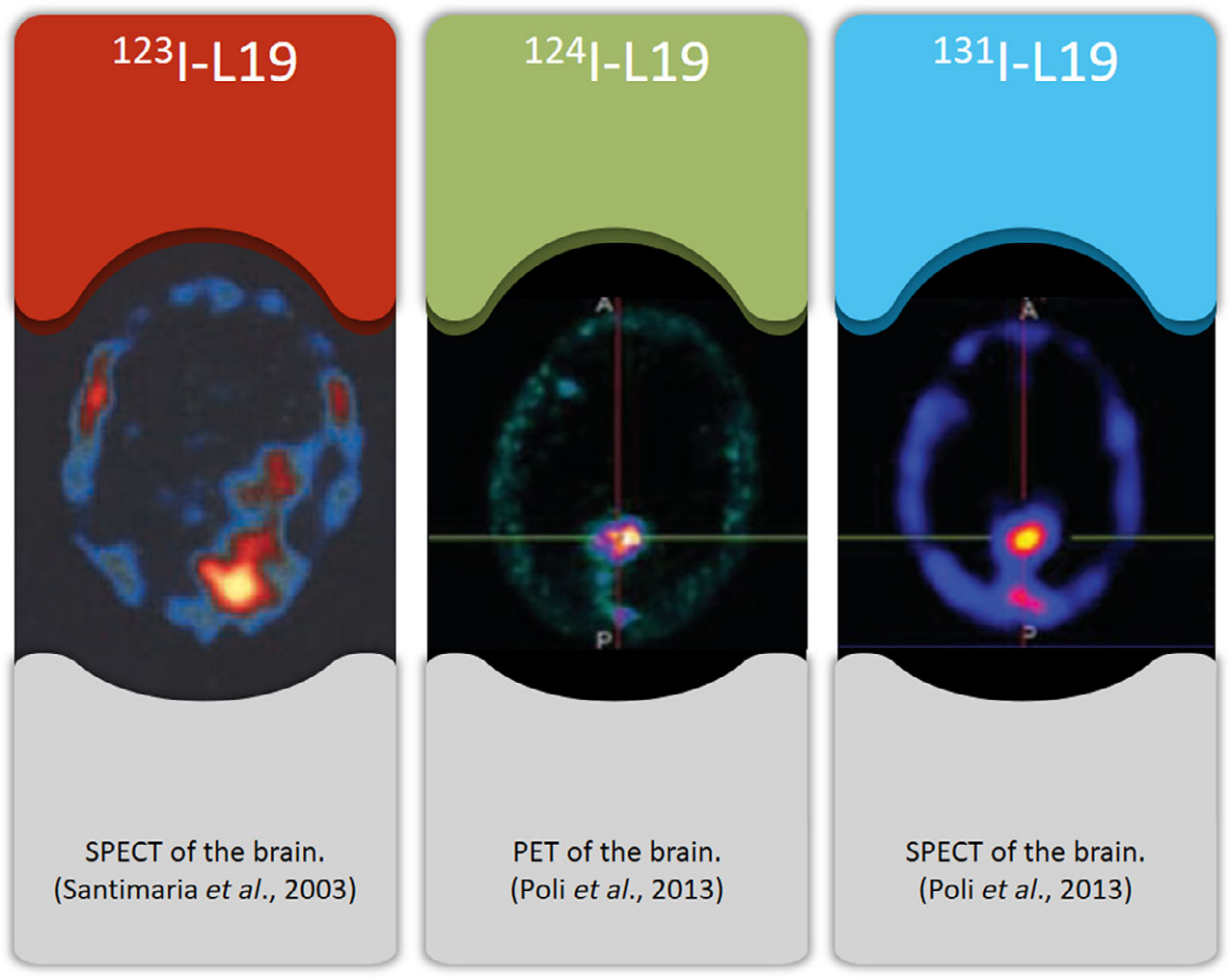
Methods to visualize ED‐B noninvasively in a patients' metastatic brain lesion. Left: SPECT scan where red/orange areas indicate uptake of ^123^I‐L19, middle: PET scan where purple/yellow area indicates uptake of ^124^I‐L19 and right: SPECT scan with uptake of ^131^I‐L19 in the red/yellow area. Images adapted from Santimaria *et al*. (2003) and Poli *et al*. (2013).
